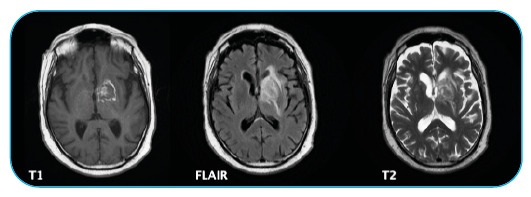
The lesion partially calcified without significant postcontrast enhancement in the left striatocapsular region. The reduction of the edema component, which does not currently extend to the temporal lobe, is more evident in T2 and FLAIR. Reduction of the mass effect on the ventricular system, with the incipient deviation of the pellucid septum to the right persisting.
